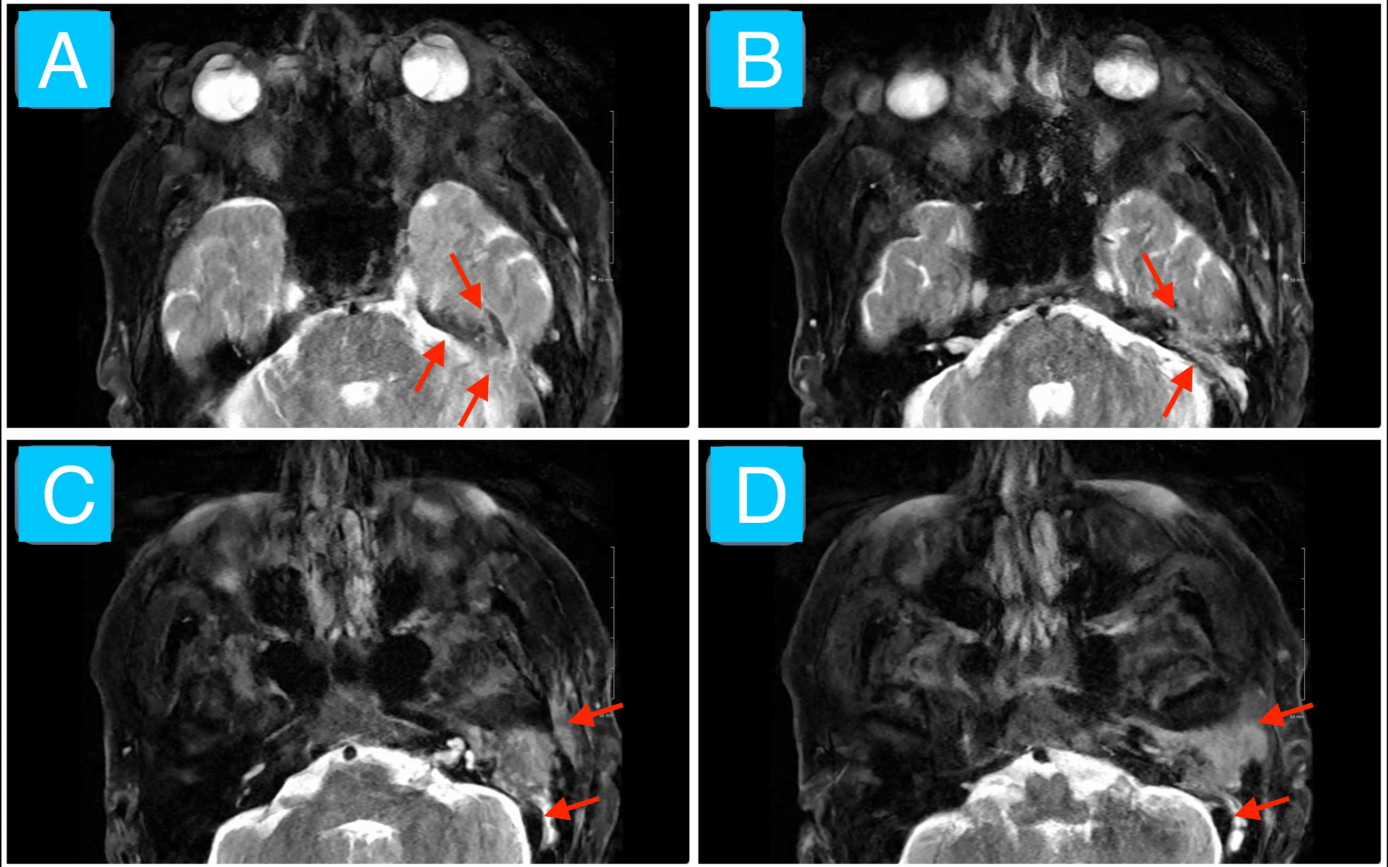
Non-contrast T2-weighted MRI of the face Axial T2-weighted sequence MRI of the face without contrast from most superior (image A) to most inferior (image D) in a 63-year-old male showing a 3.6 × 1.8 × 3.0 cm poorly defined, mostly homogeneous, hyperintense lesion invading the left EAC, middle ear, petrous apex, mastoid bone, posterior TMJ wall, temporal lobe, facial nerve canal, and retromandibular space (red arrows). These findings are consistent with an aggressive mass despite motion artifact. Biopsy confirmed moderately differentiated SCC. EAC: external auditory canal; TMJ: temporomandibular joint; SCC: squamous cell carcinoma
